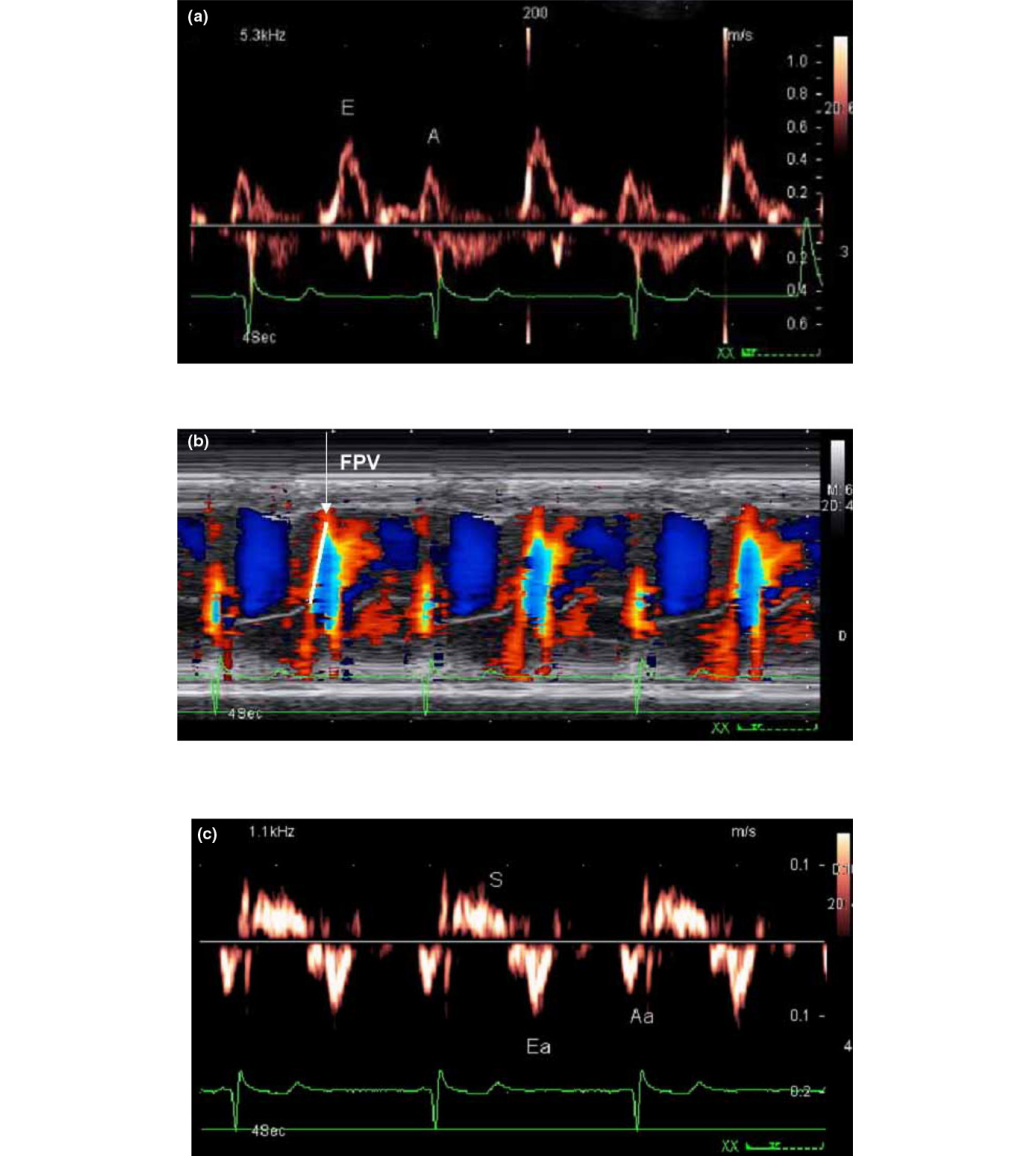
Echocardiographic parameters to estimate LV filling pressures (a) Mitral inflow, (b) color M-mode-derived flow propagation velocity (FPV), and (c) Tissue Doppler velocities at the lateral corners of the mitral annulus
